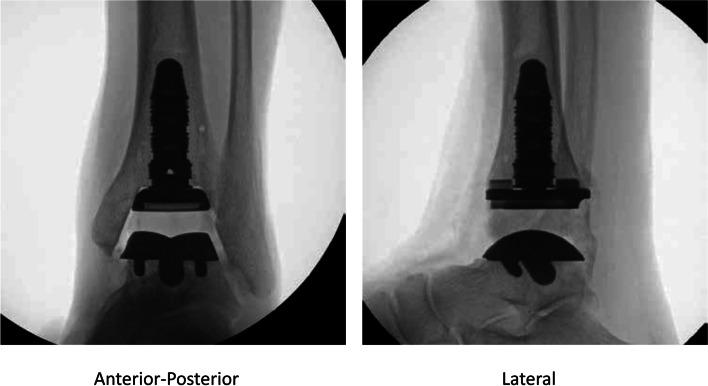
X-ray images of the INBONE II implant system. Intra-operative anterior-posterior and lateral fluoroscopic images of the ankle with INBONE II implant system. (Image courtesy of Wright Medical Technology, Inc., Memphis, TN, US)
